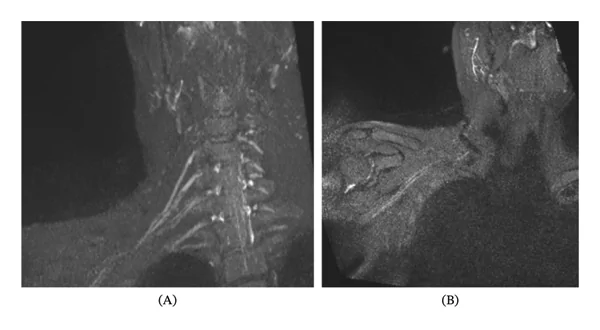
Coronal 3‐D STIR MRI of the right brachial plexus demonstrating no evidence of focal enhancement, dilatation, or hyperintensity within the brachial plexus to explain the patient’s right upper extremity deficits. An unremarkable supraclavicular plexus with trunk formation (A) and infraclavicular plexus with cords leading to terminal branching past the coracoid process in the subpectoral space (B) are depicted.
